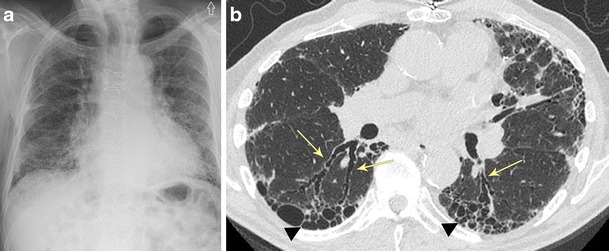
Bronchiectasis due to usual interstitial pneumonia (UIP). a Frontal chest x-ray showing prominent coarse interstitial and reticular opacities with a peripheral and basilar predominance, findings consistent with a UIP pattern of fibrosis. b Axial CT imaging demonstrating the basilar-predominant varicoid traction bronchiectasis (*arrows*) associated with honeycombing (*arrowhead*) and subpleural reticulation
